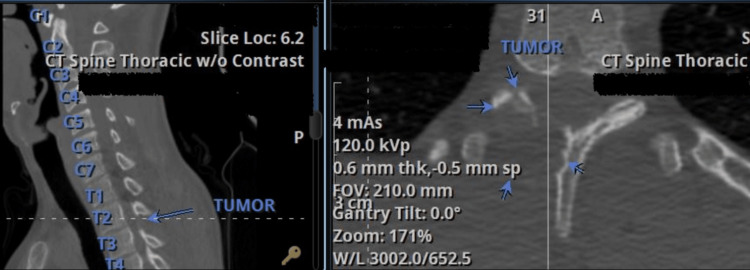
Contrast-enhanced CT showing an osteolytic lesion centered in the right T2 lamina and inferior facet, with slight extension into the right neural foramen near the exiting T2 nerve root.
